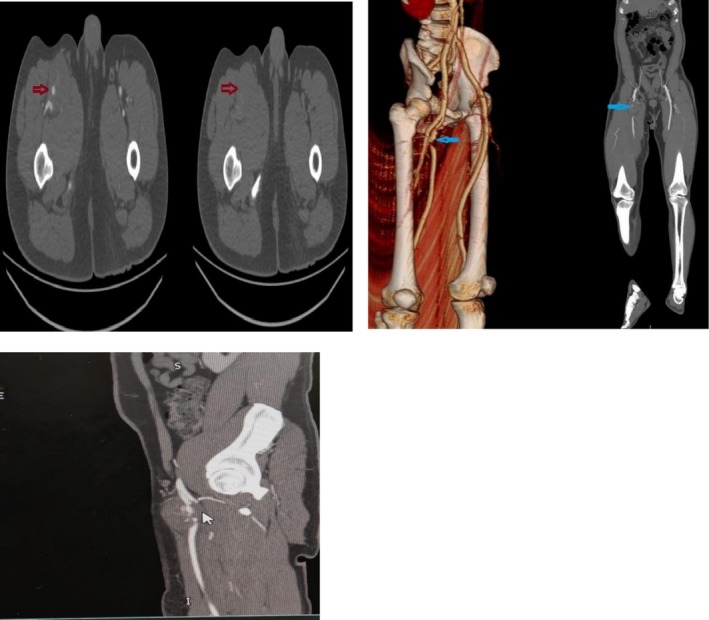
Computed tomography of lower limb: A 46 mm focal segment of the superficial femoral artery about 36 mm from its origin shows significant irregular luminal narrowing and mild posterior displacement. A 67 × 45 mm hematoma is noted around this segment predominantly along the anterior aspect with contrast ooze noted within.
